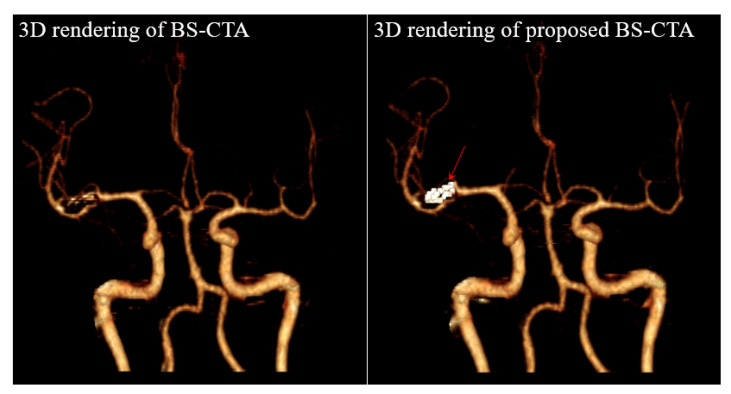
Results of 3D rendering of conventional BS-CTA (left) and proposed BS-CTA (right).
